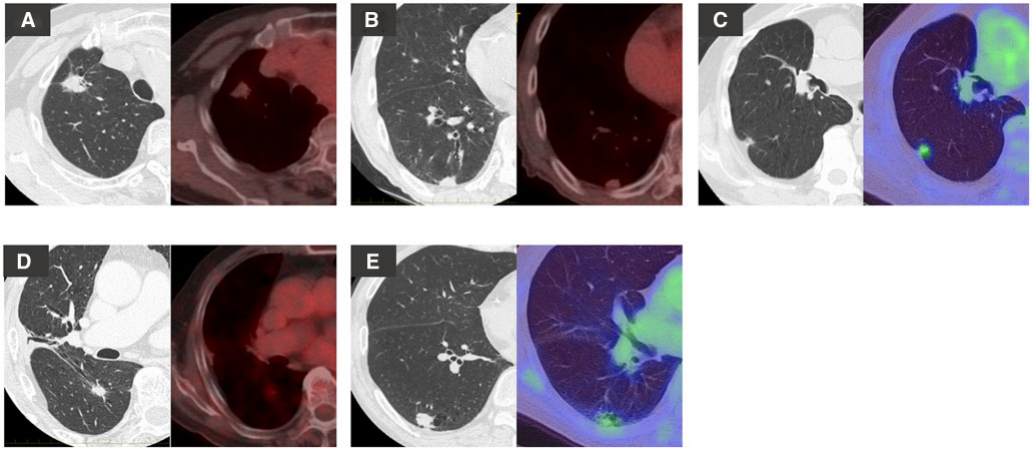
Preoperative computed tomography and [18F]-fluoro-2-deoxy-D-glucose-positron emission tomography/computed tomography images of (A) Case 1, (B) Case 2, (C) Case 3, (D) Case 4 and (E) Case 5 as presented in Table 2.
